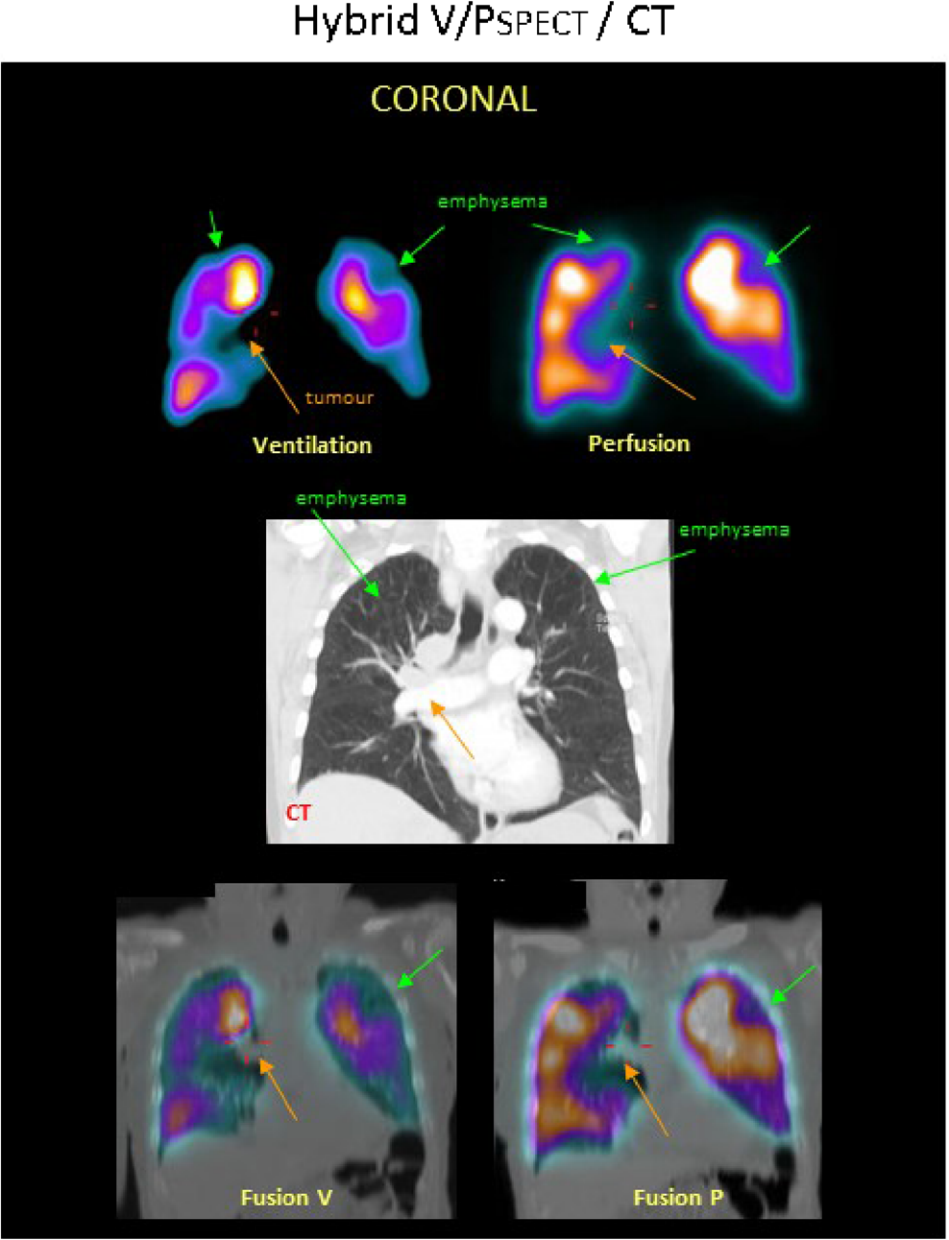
A patient with COPD, emphysema and tumour. Coronal slices display uneven distribution of ventilation with a pattern of deposition of ^99m^Tc-Technegas® that is typical for COPD. Perfusion follows the ventilation pattern. Matched ventilation and perfusion defects are observed in both upper lobes (green arrows) and to the right of the mediastinum (orange arrows). In the medial row of the corresponding coronal CT slice, emphysema is seen in both upper lobes (green arrows), as is a tumour in the mediastinum (orange arrow). Fusion images of CT and ventilation SPECT and CT and perfusion SPECT are shown
